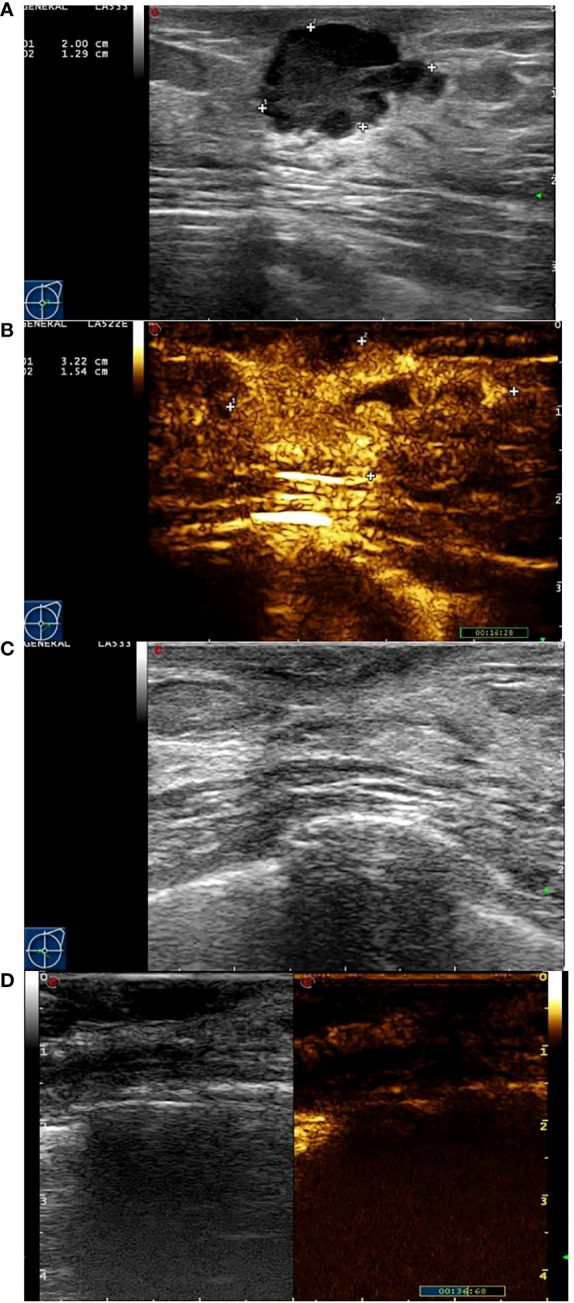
Images from a patient of response to NAC. (A) At baseline before NAC, the tumor size was measured by ultrasonography. (B) At baseline before NAC, the tumor size was measured by contrast-enhanced ultrasound. The extent of tumor was significantly larger than that of ultrasonography. (C) After the last cycle of NAC before surgery, the tumor has disappeared fundamentally by ultrasonography. (D) After the last cycle of NAC before surgery, there was not area of high enhancement fundamentally.
